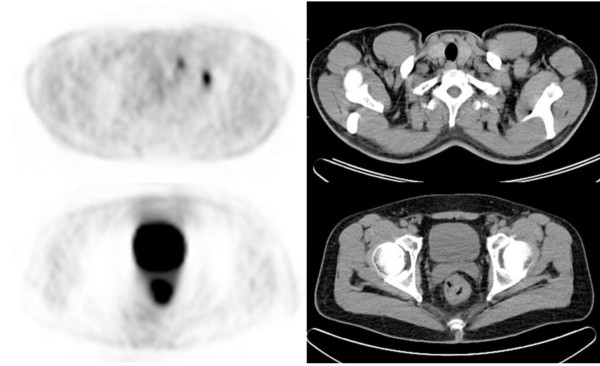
Appearance of primary tumor and left supraclavicular lymph node metastases in PET/CT image.
